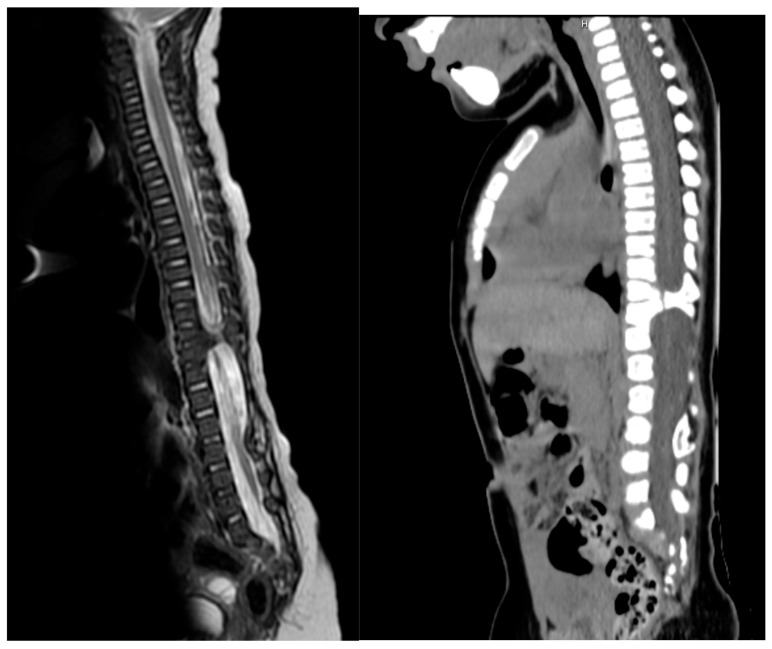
Split cord malformation (SCM) type I due to vertebral bony spur, as seen on sagittal T2W-MR on the left and on sagittal CT-scan on the right side.
